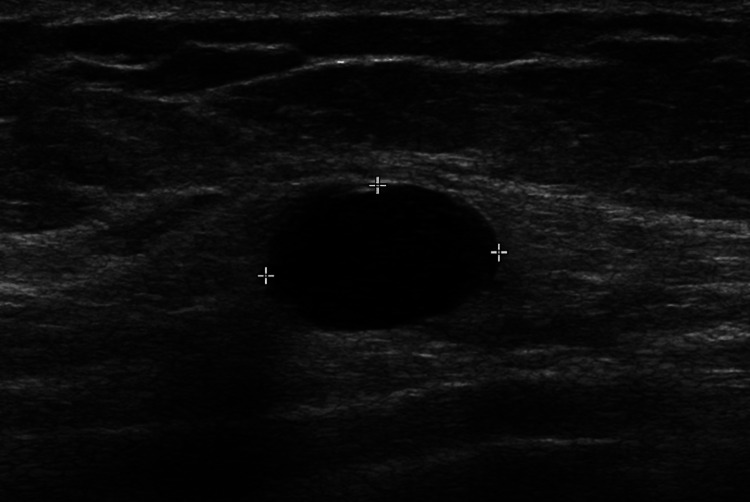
Targeted left breast ultrasound Grayscale ultrasound of the left breast demonstrates a 2 cm oval, well-circumscribed hypoechoic lesion with parallel orientation and smooth margins, consistent with a benign BI-RADS 3 finding in a young post-radiation survivor.
